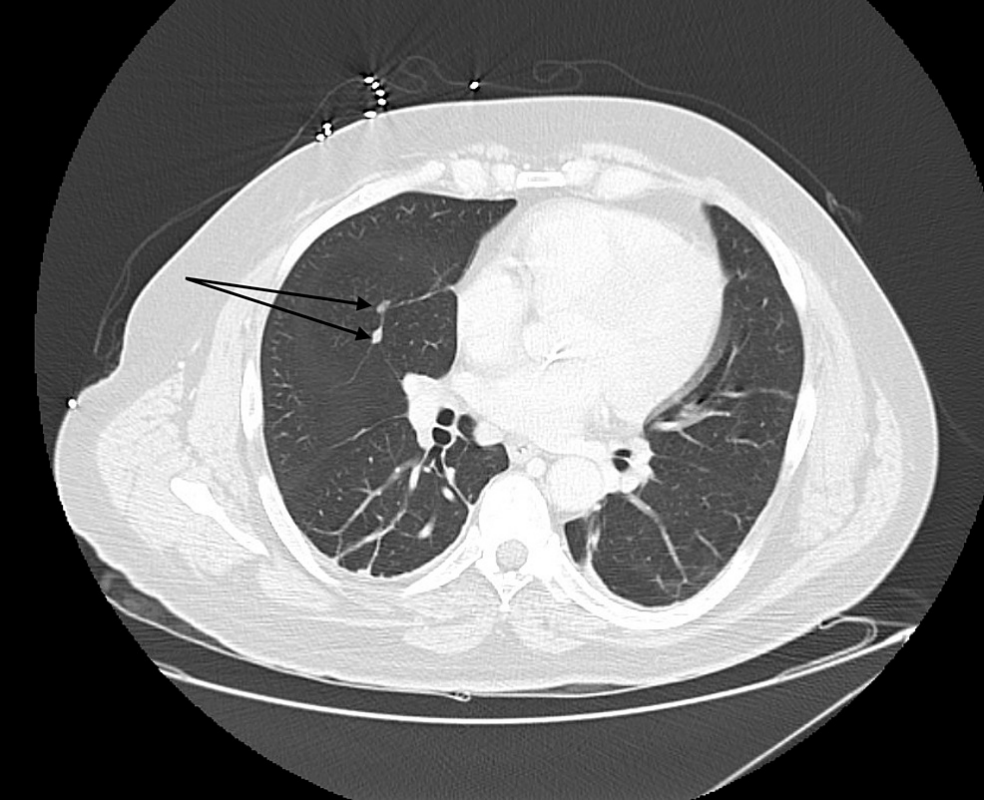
CT of the chest showing two lung nodules in the right middle lobe (black arrows)
